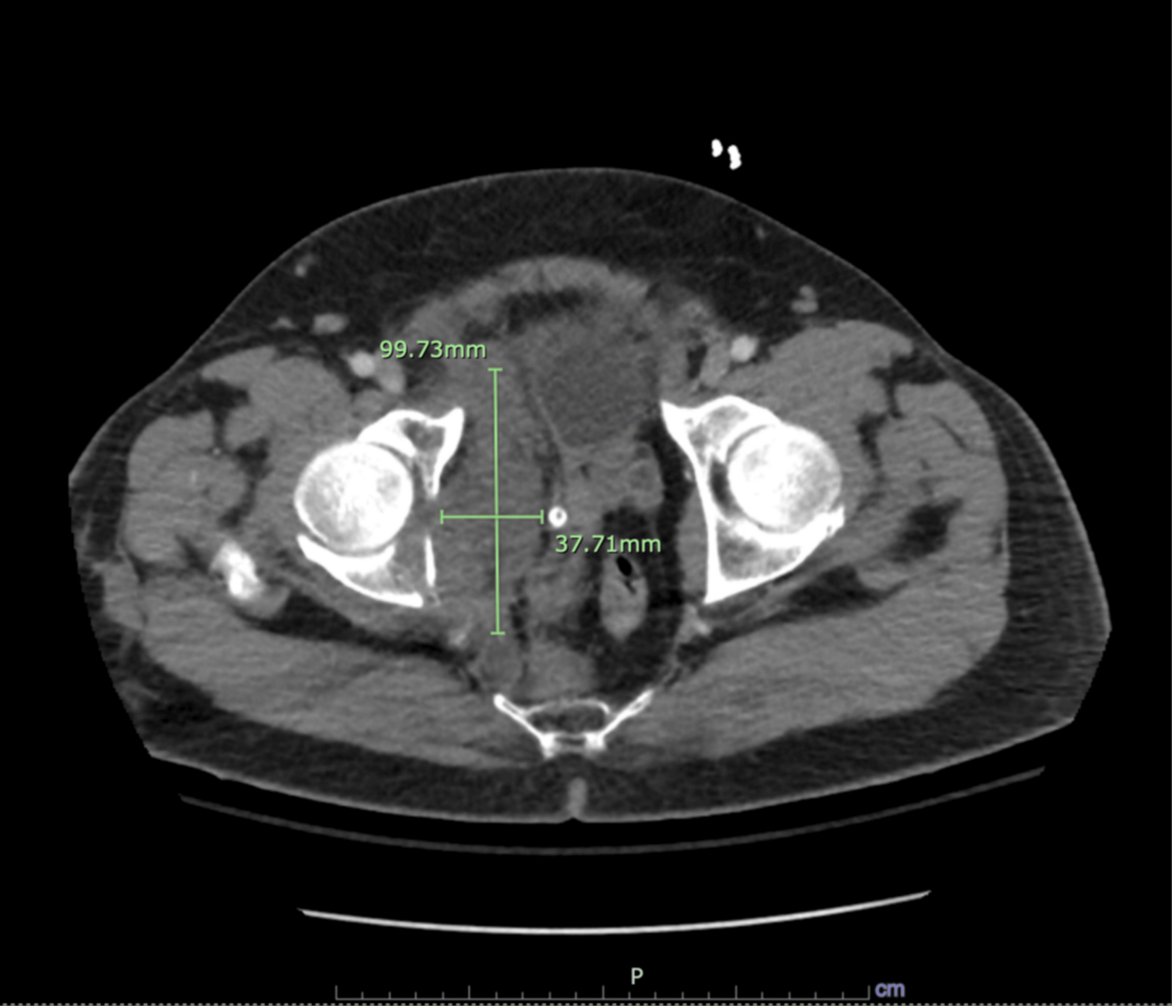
CT scan showing acute thoracic aortic injury, pseudoaneurysm, and associated periaortic hematoma.
